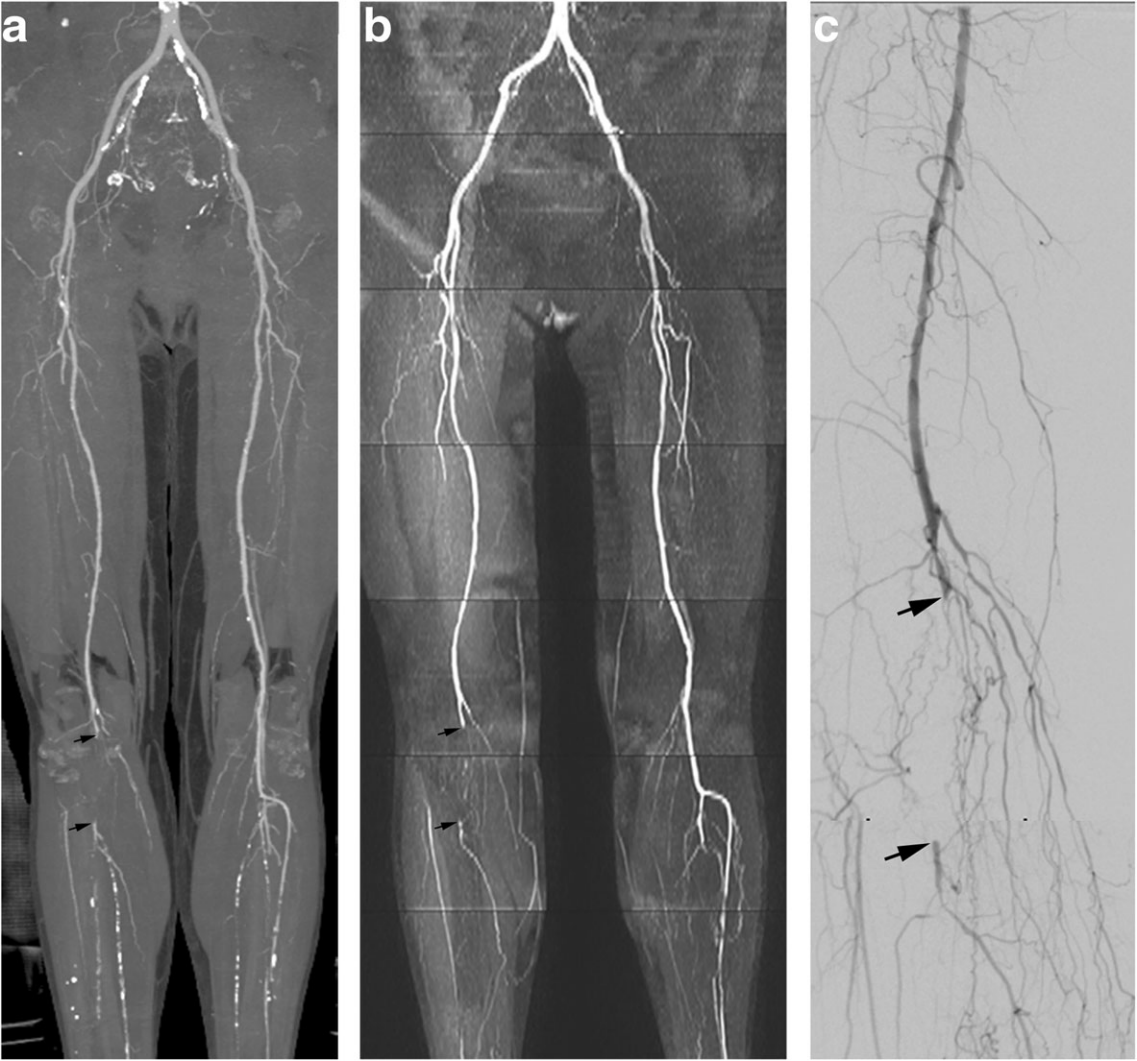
a Multiple stenoses at right femoral artery and occlusion (*arrows*) of right popliteal artery were shown with CTA image; however, occlusions at bilateral lower leg were difficult to identify duo to multiple calcified plaques. b Occlusion of the right popliteal artery was also shown with QISS (*arrows*). Calcified plaques were not problematic with QISS, with occlusions easily identified at calf. c Occlusion of the right popliteal artery was proved by DSA (*arrows*). More collateral circulation vessels were shown with DSA
